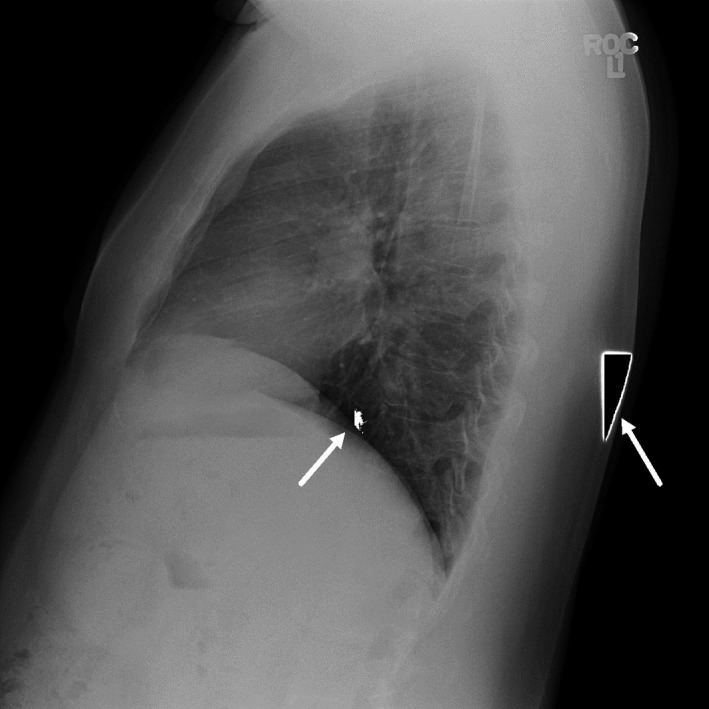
LAT chest radiograph showing artifacts (white arrows) that appeared suddenly, necessitating a detector replacement on August 5, 2014.
